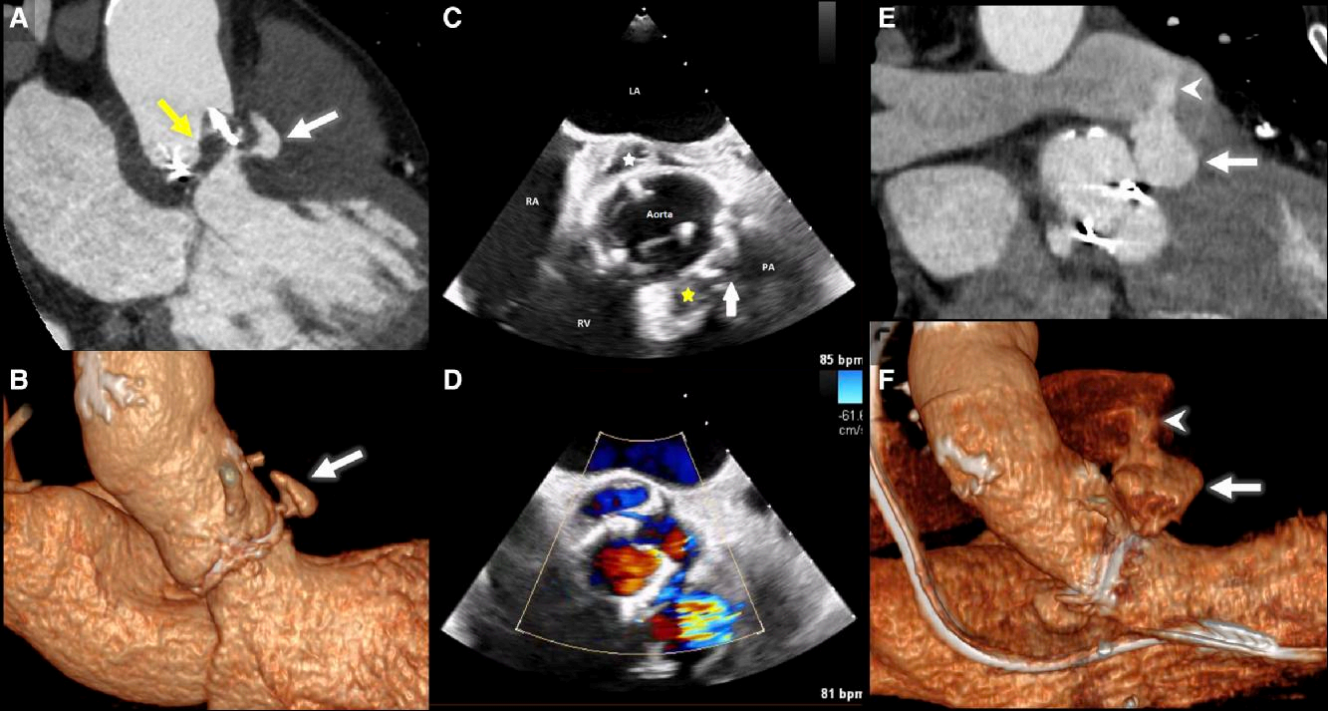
Imaging of prosthetic valve endocarditis complicated by pseudoaneurysm and aortopulmonary fistula. Contrast-enhanced CT scan (CT angiography) (*A*) and 3D virtual reconstruction (3D VR) (*B*) showing hypoattenuated leaflet thickening (yellow arrow) and a contained pseudoaneurysm (white arrow) upon admission. Cardiac transoesophageal echocardiogram in B-mode (*C*) and Doppler mode (*D*) of the aortic valve acquired post-operatively, revealing pseudoaneurysm (yellow star) with evidence of fistulization to the pulmonary trunk (white arrow). A second, smaller pseudoaneurysm is also visible on the opposite side of the valve (white star). Postoperative CT angiography (*E*) and 3D virtual reconstruction (*F*) showing rapid progression in size of the pseudoaneurysm (white arrow) with new-onset fistula to the pulmonary trunk (white arrowhead). LA = left atrium, PA = pulmonary artery, RA = right atrium, RV = right ventricle.
